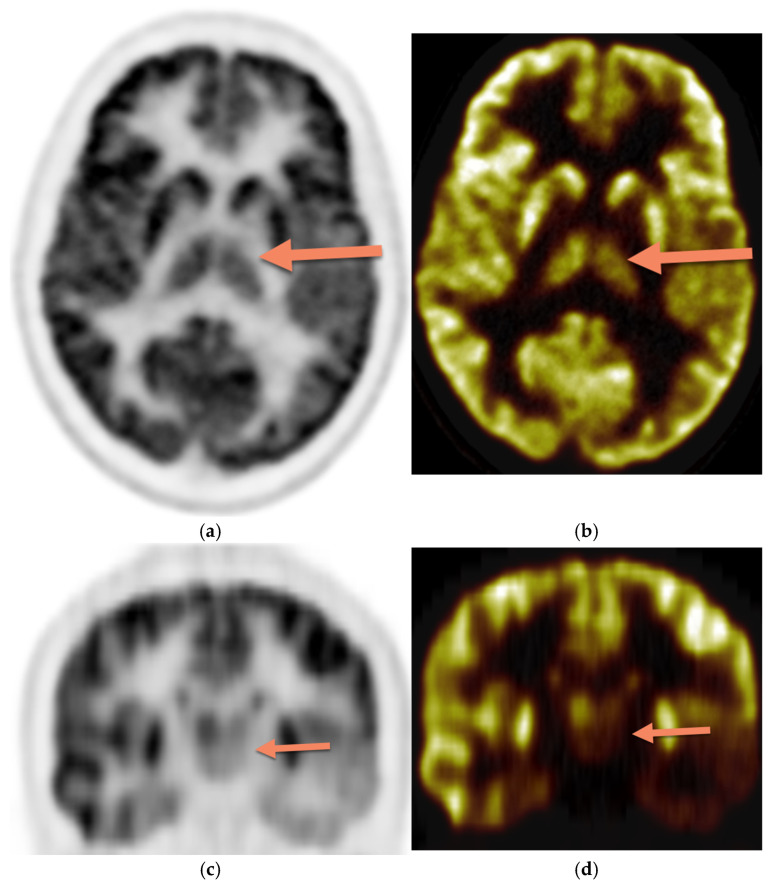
Brain 18F-FDG PET, (a) (sagittal grey scale), (b) (sagittal rainbow color), (c) (coronal grey scale), and (d) (coronal rainbow color) in a 13-year-old female with asymmetric decreased activity in the left temporal lobe. The uptake of the left thalamus is also reduced (arrow) compared with the contralateral side.
